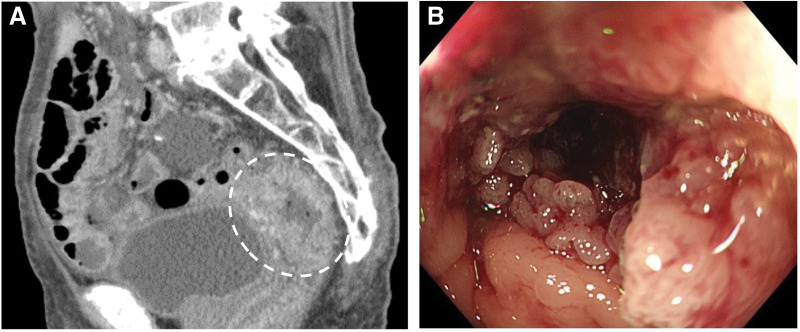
Preoperative imaging studies. (A) Contrast-enhanced pelvic CT shows a 40-mm segment of wall thickening with enhancement in the rectosigmoid region. (B) Colonoscopic examination reveals a type 2 tumor occupying approximately three-fourths of the lumen circumference, located 20 cm from the anal verge.
